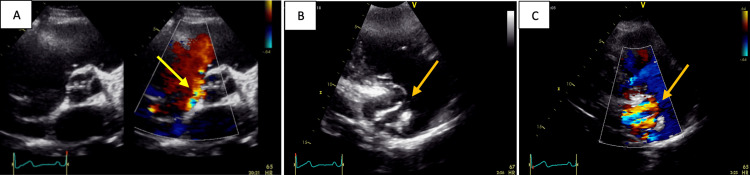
A: Parasternal short axis view showing on color Doppler a systolodiastolic flow originating from the aorta and moving toward the right atrium (yellow arrow). B, C: Modified parasternal long-axis view focused on the right cavities showing a small canal at the wall of the right atrium with aliased flow (orange arrows)
